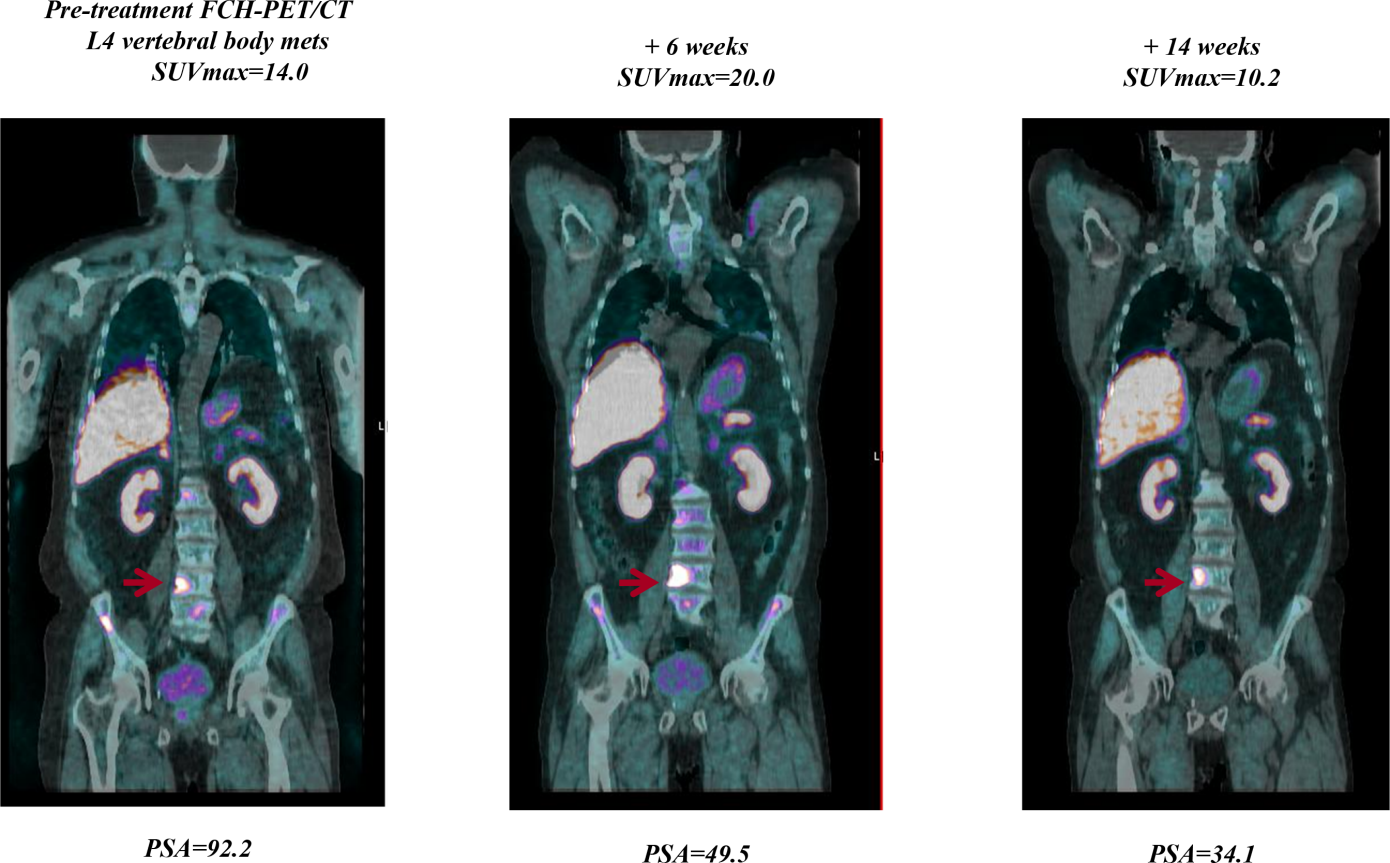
Example of a patient with a rapidly declining PSA level but a week 6 FCH-PET/CT being read as progression in bone lesions By week 14, this progression improved, indicating that the progression seen at week 6 was due to bone flare.
